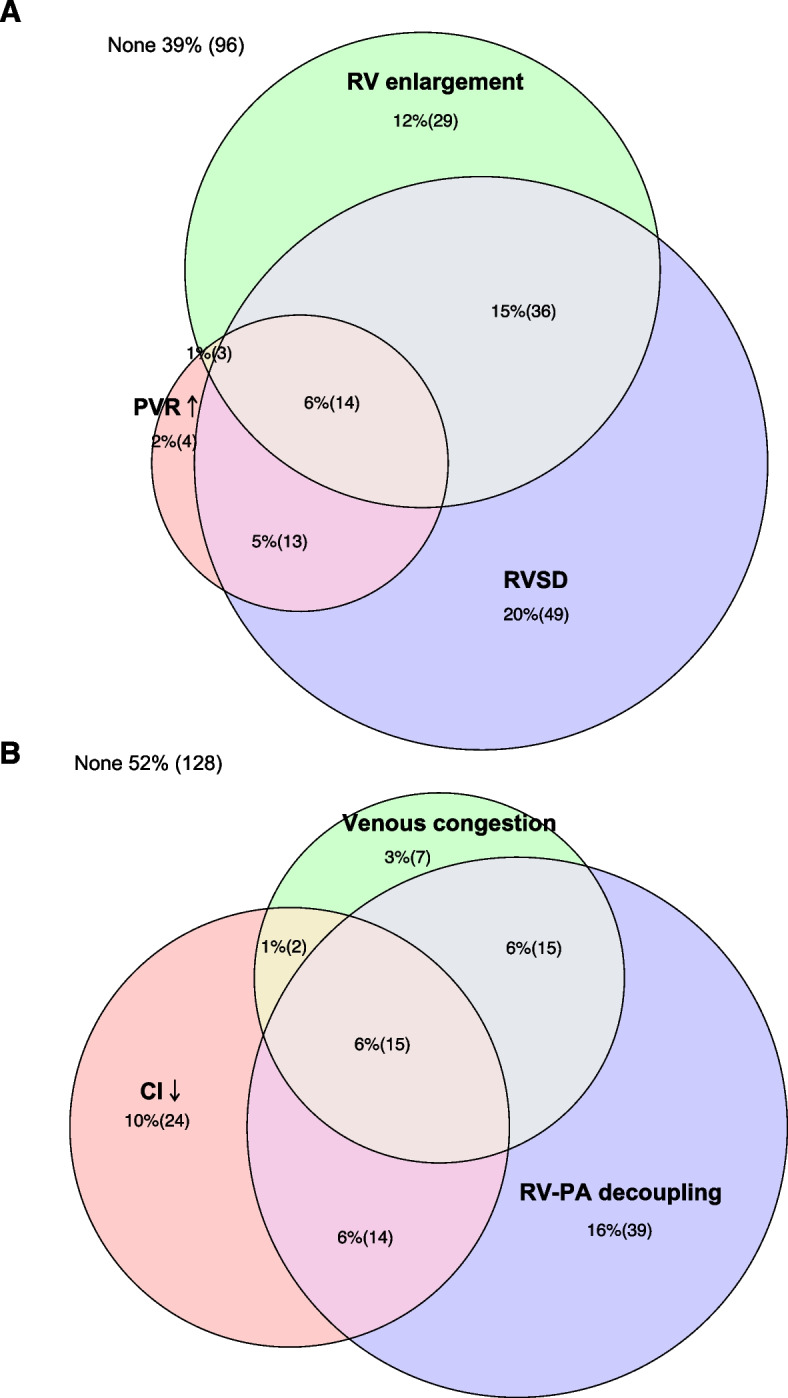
Distribution of haemodynamic and echocardiographic parameters. A The distribution of RV-related echocardiographic parameters, demonstrating frequent overlap of RV enlargement, RVSD and PVR increase. B The distribution of haemodynamic outcomes, demonstrating frequent overlap of systemic venous congestion, RV-PA decoupling and low cardiac index. TAPSE: tricuspid annular plane systolic excursion; FAC: fractional area change; S’: tissue Doppler peak velocity of tricuspid annulus; RVSD: right ventricular systolic dysfunction; PVR: pulmonary vascular resistance; RV-PA: right ventricle-pulmonary artery; CI: cardiac index
